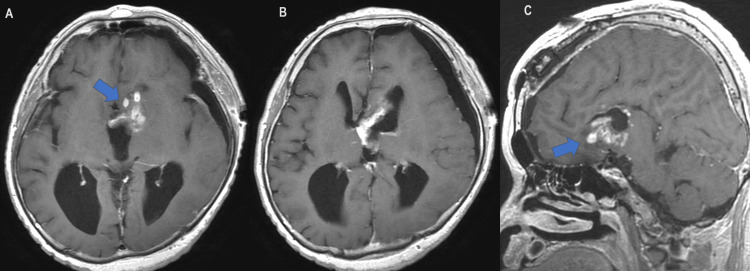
Postoperative MRI of the patient. Contrast-enhanced T1-weighted images display a reduced tumor in the ventricles (A and B) and improved hydrocephalus, with the mass's decreased size more clearly indicating its left frontal basal origin (A and C; arrows).
